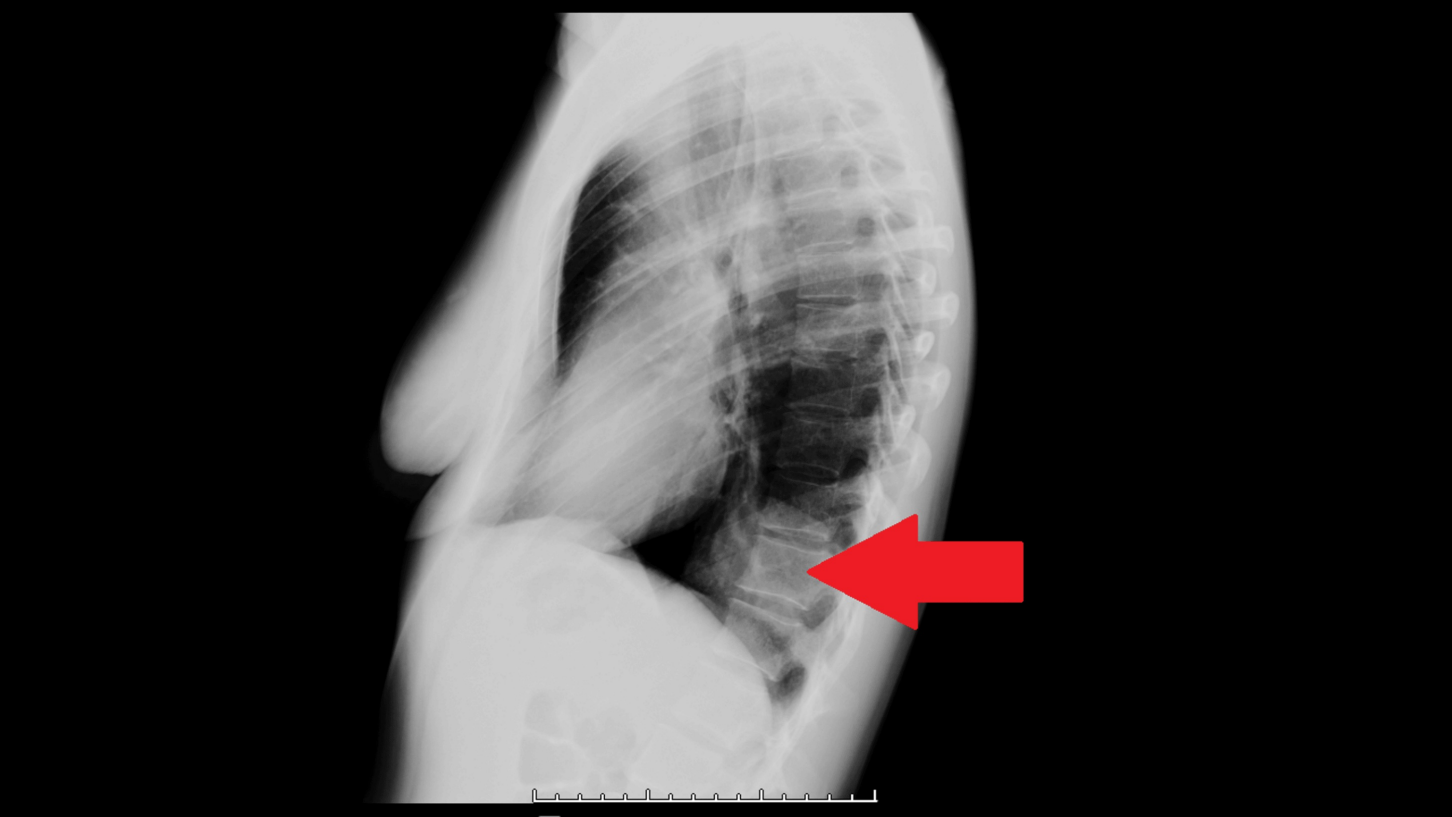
Left lateral chest X-ray obtained prior to the transthoracic biopsy The lesion is marked with the red arrow.
